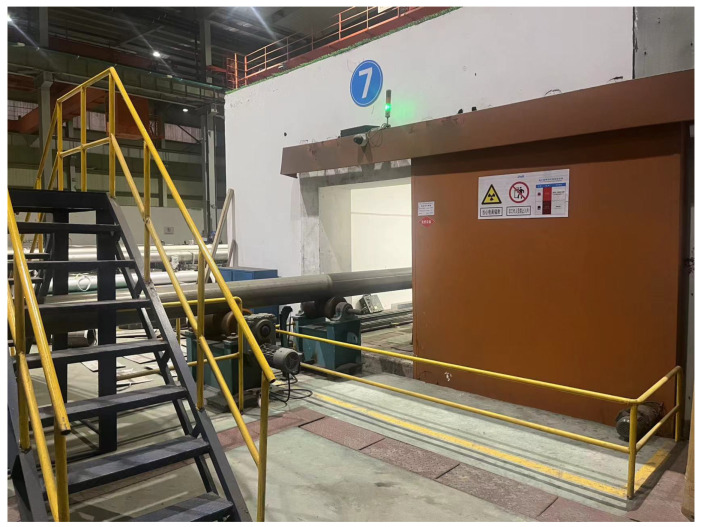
X-ray equipment. The transport device can automatically transport the steel pipes to the shooting room and automatically transport them out after the shooting is completed.
